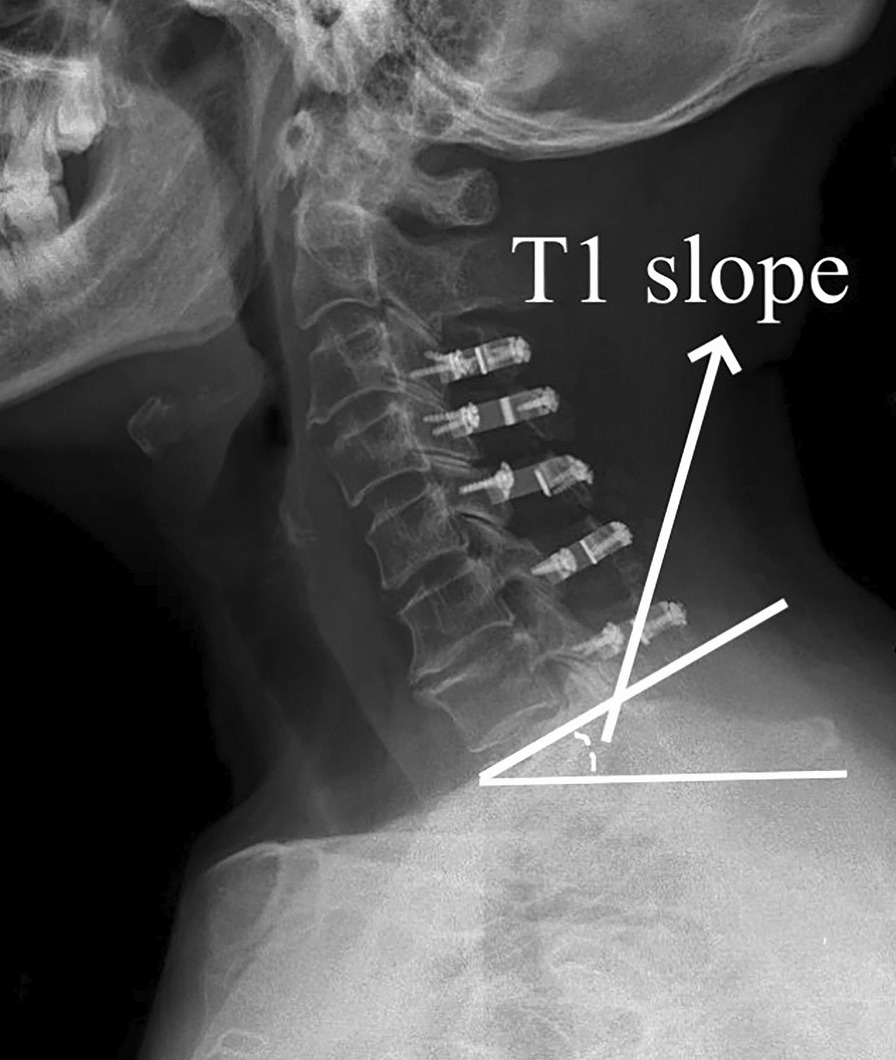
LP postoperative radiographs, which include measurements of the T1 slope (the angle between parallel end plate lines and horizontal reference lines on T1)
